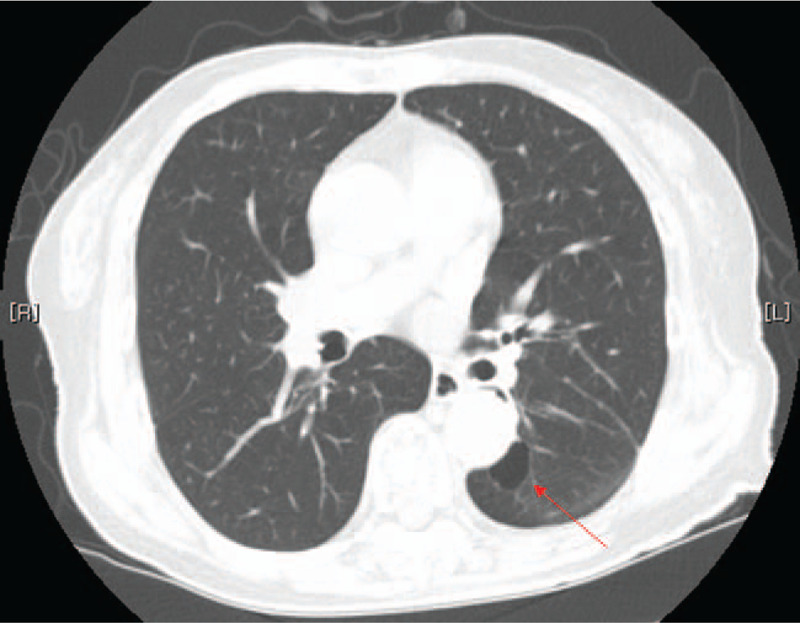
A follow-up chest computed tomography scan on July 31, 2015 revealed that the primary tumor had changed to a thin-walled cavitation.
